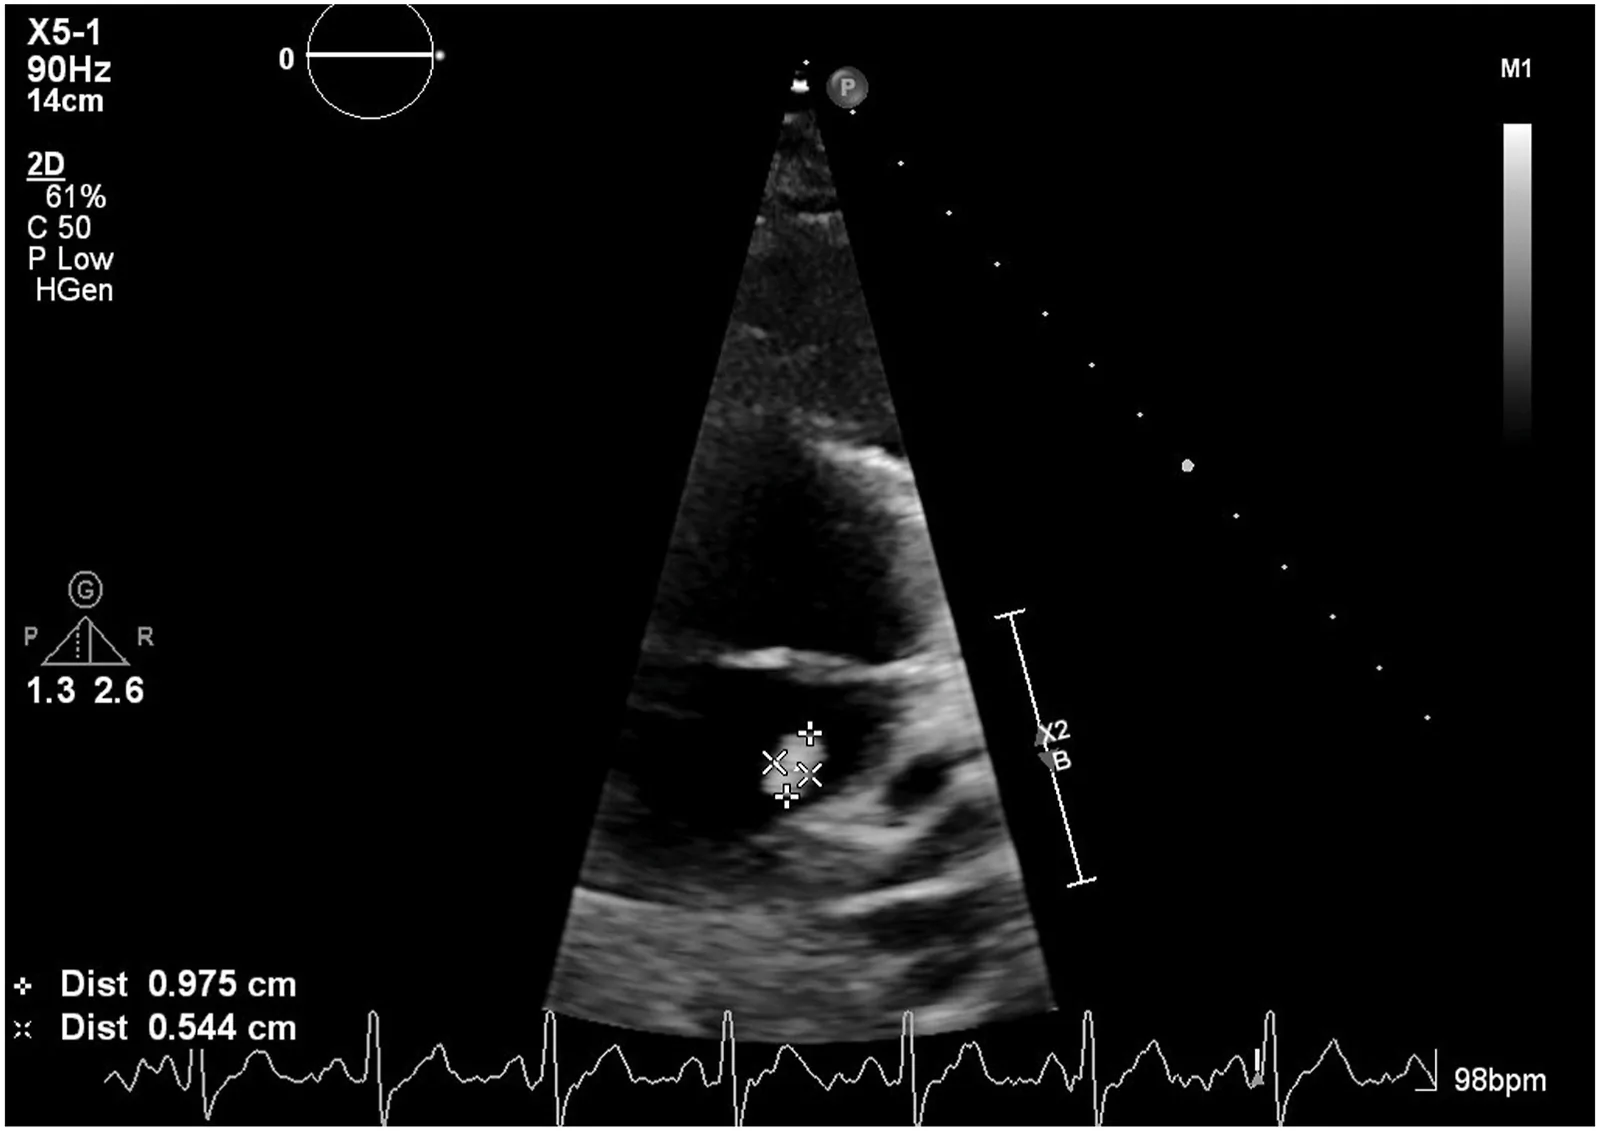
Two-dimensional TTE, zoomed parasternal long-axis systolic view, demonstrates an irregular, echogenic mass (9.8 × 5.4 mm) attached to the atrial surface of the MV leaflet, consistent with a vegetation.
